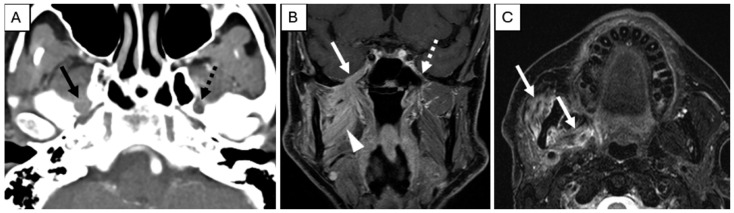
Pre-treatment Examination: Perineural Tumor Spread. (A), Axial contrast-enhanced computed tomography of the skull base demonstrates loss of perineural fat and thickening of the mandibular division of the right trigeminal nerve (solid arrow). Note the preserved fat plane on the left (dashed arrow). (B), Coronal T1-weighted, fat-saturated postcontrast image demonstrates abnormal thickening and enhancement of the right mandibular division of the trigeminal nerve extending through the foramen ovale into the cavernous sinus (solid arrow). Note the normal left side (dashed arrow). Abnormal enhancement of the right masticator space muscles (arrowhead) is compatible with subacute denervation changes. (C), Axial T2-weighted image demonstrates hyperintense signal within the right muscles of mastication (arrows) compatible with subacute denervation changes, directing the search pattern to the mandibular division of the trigeminal nerve.
